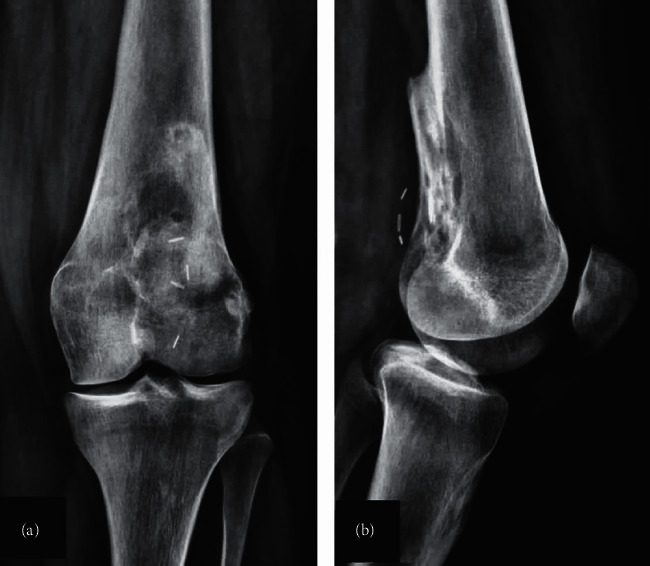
Parosteal osteosarcoma (PAO) in a 16-year-old female (no. 6). Anteroposterior (a) and lateral (b) radiographs four years after operation showing recurrence of PAO.
